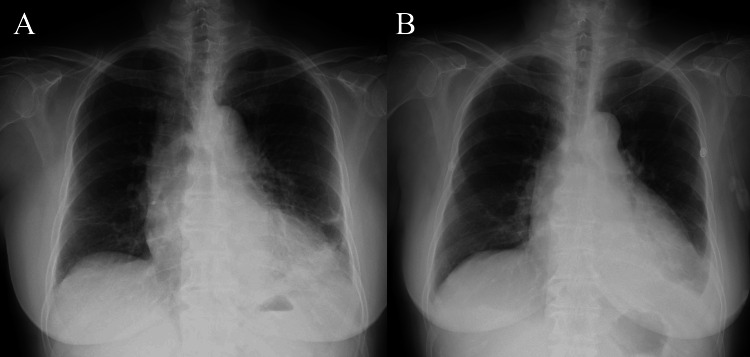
Chest X-rays on admission and on day 17 (A) Chest X-ray on admission showing cardiomegaly and decreased transparency in the bilateral lower lung fields. (B) Chest X-ray on day 17 showing slightly improved transparency in the bilateral lower lung fields compared with admission.
